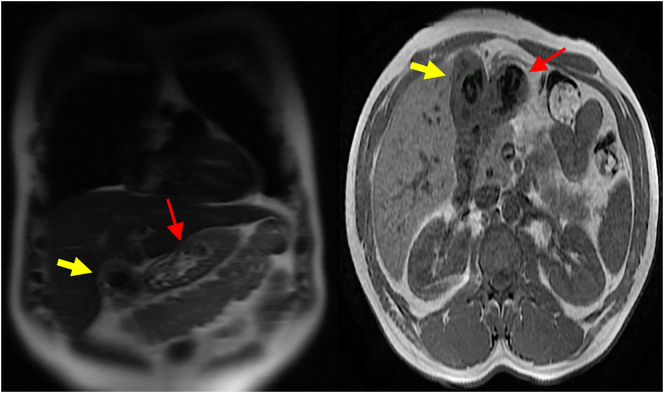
MRI view showing diffuse parietal thickening of the gallbladder (yellow arrow) with multiple cystic pockets and invasion of the antropyloric region of the stomach (red arrow).
